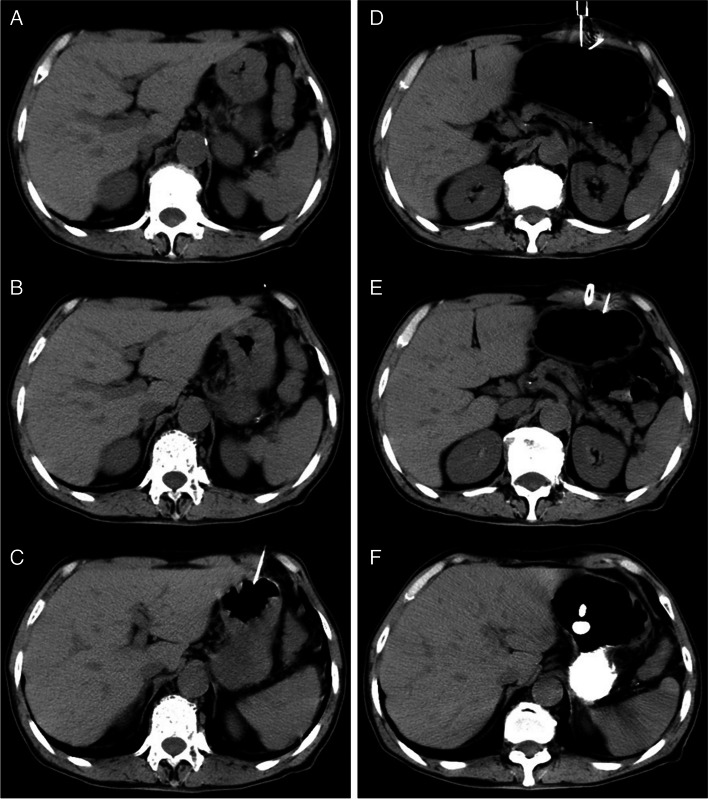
A 56-year old man who cannot underwent the gastrostomy under DSA guided due to the esophagus complete obstruction was referred to our department to undergo a CT guided percutaneous gastrostomy for long-term intragastric feeding. A A prepared abdominal CT scan was performed to localize the stomach and design the puncture pathway. B A metallic marker was placed on the skin surface to show the puncture pathway. C A 21-gauge Chiba needle was introduced into the gastric lumen and then the air inflation of gastric lumen was performed through the 21-gauge Chiba puncture needle. D After the stomach lumen insufflations finished, puncture procedure was performed with the gastropexy device. The complete gastropexy device was removed, maintaining traction on the wire loop retrieving the suture, which was released and tied in a standard surgical knot, opposing the anterior stomach to the anterior abdominal wall. E After successful gastropexy at both marked points a stab incision of about 5 mm was performed in between to insert the trocar with peel-away sheath carefully under CT guidance. The CT scan showed the trocar with peel-away sheath and the 21 G Chiba needle. F Once correct position was confirmed the trocar was removed and the balloon tube was inserted into the gastric lumen. The CT scan showed the balloon tube filled with 3 ml of contrast medium through an extra valve on the side using a syringe.
